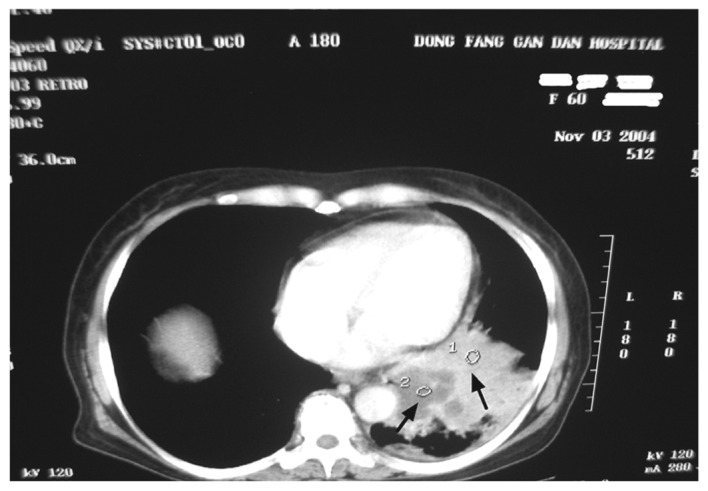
Computed tomography of the abdomen shows possible choledochoduodenal fistulas in the common bile duct (arrows 1 and 2).
